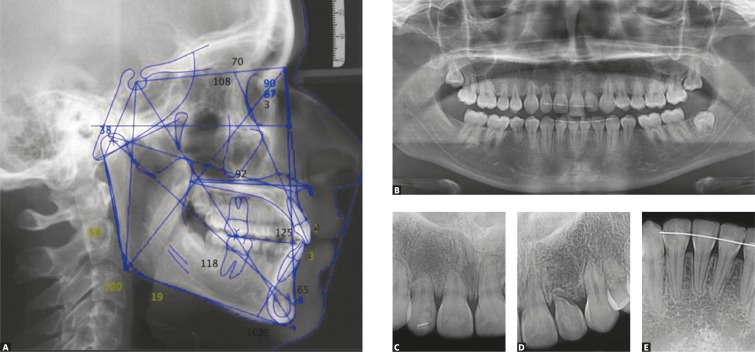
Post-treatment radiographs. A) Cephalometric tracing; B) Panoramic
